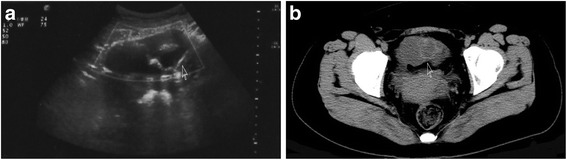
a Ultrasound graph revealed echogenic foci and linear reticular echogenic structures. b CT scan revealed a lower abdominal cystic mass with hyperdense linear components
